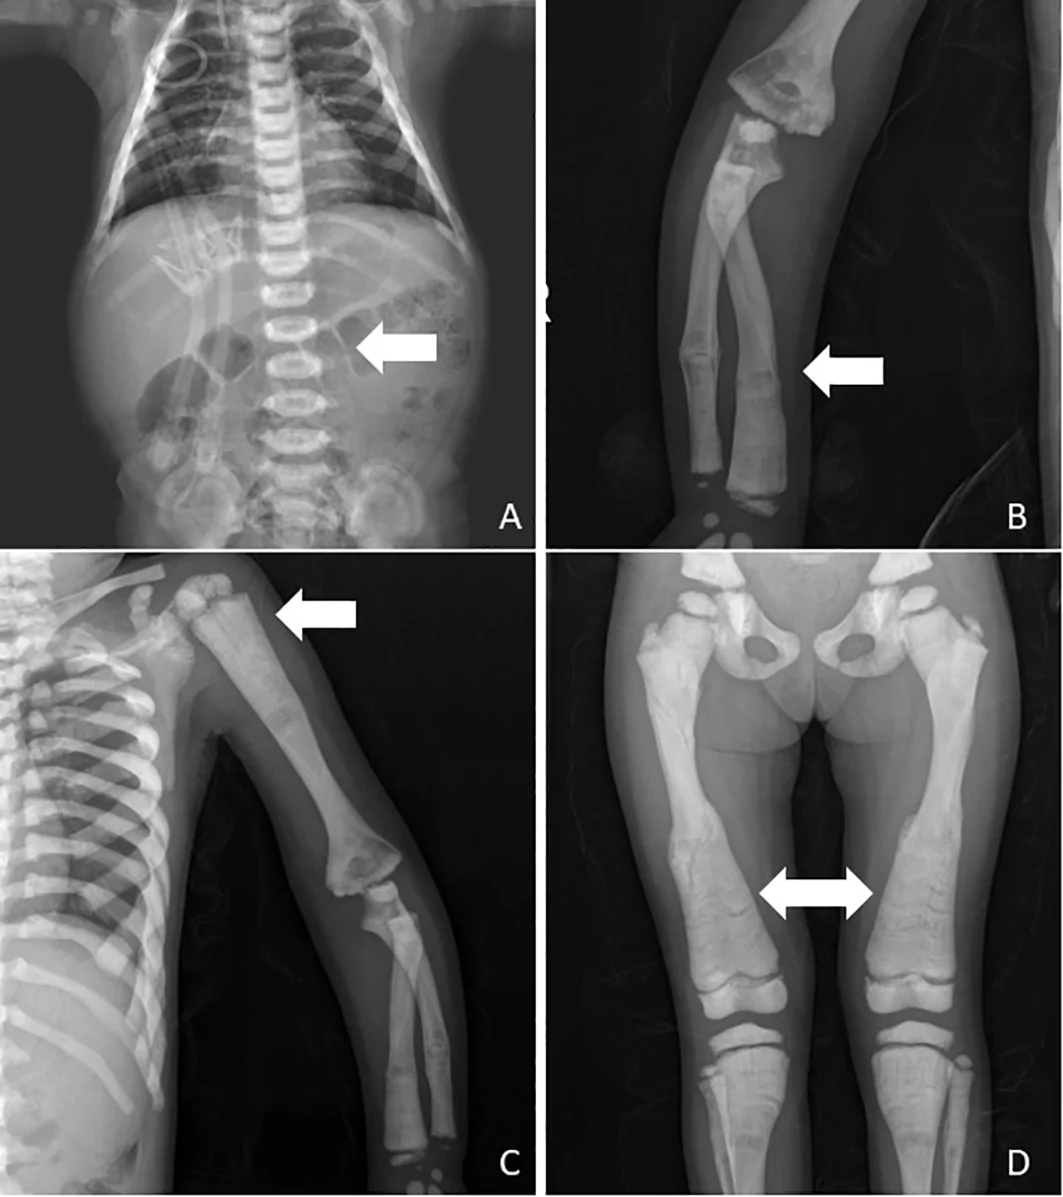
Representative radiographic findings of osteopetrosis. Panel (A) shows generalized osteosclerosis with possible vertebral endplate sclerosis, panel (B) shows fracture-related callus formation, panel (C) shows metaphyseal widening, and panel (D) hows Erlenmeyer flask deformity.
